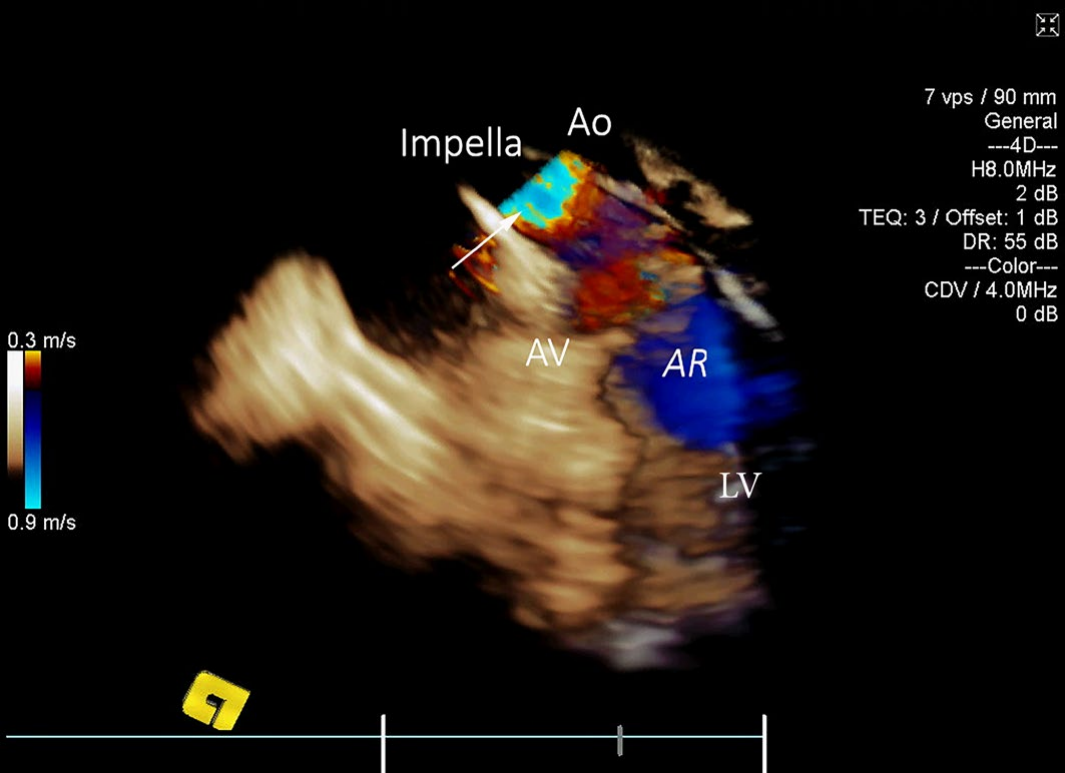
3D ICE Colour Doppler applied over the proximal ascending aorta (Ao), aortic valve (AV) and left ventricular outflow tract. Highly turbulent flow (white arrow) confirms the position of Impella CP outflow. Mild peri-catheter aortic incompetence (AR) was noted. L*V *left ventricle.
